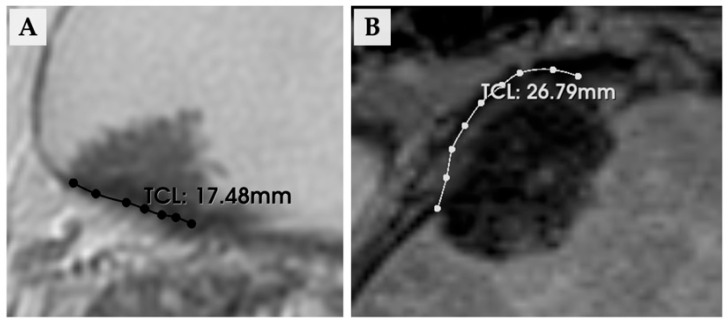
Representative examples of tumor contact length (TCL) measurement on axial T2-weighted magnetic resonance images. (A) A 64-year-old man with a TCL of 17.48 mm; pathological diagnosis was pTa. (B) A 69-year-old woman with a TCL of 26.79 mm; pathological diagnosis was pT2. Abbreviations: TCL, tumor contact length.
